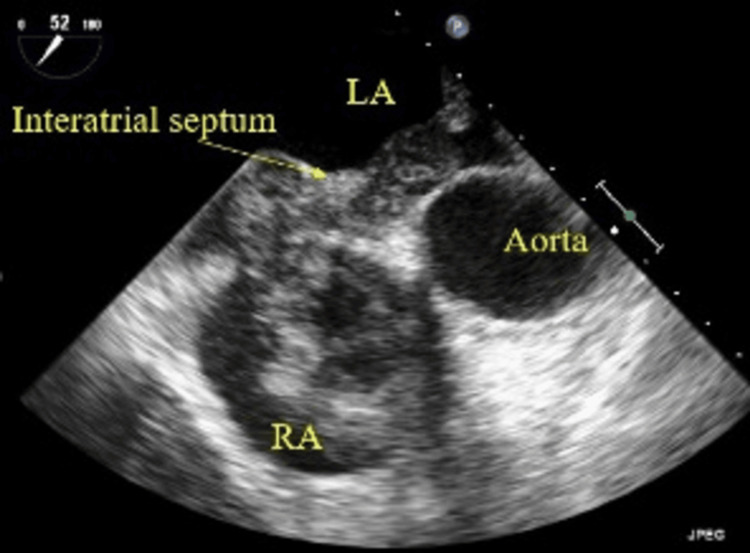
Transesophageal echocardiogram showing a mass in the RA LA, left atrium; RA, right atrium
